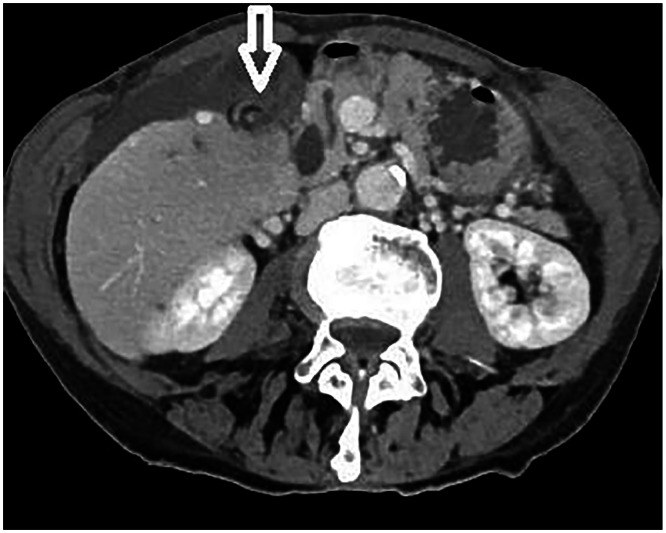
Portal venous phase CT showing the whirl sign (white arrow) indicative of gallbladder torsion.
